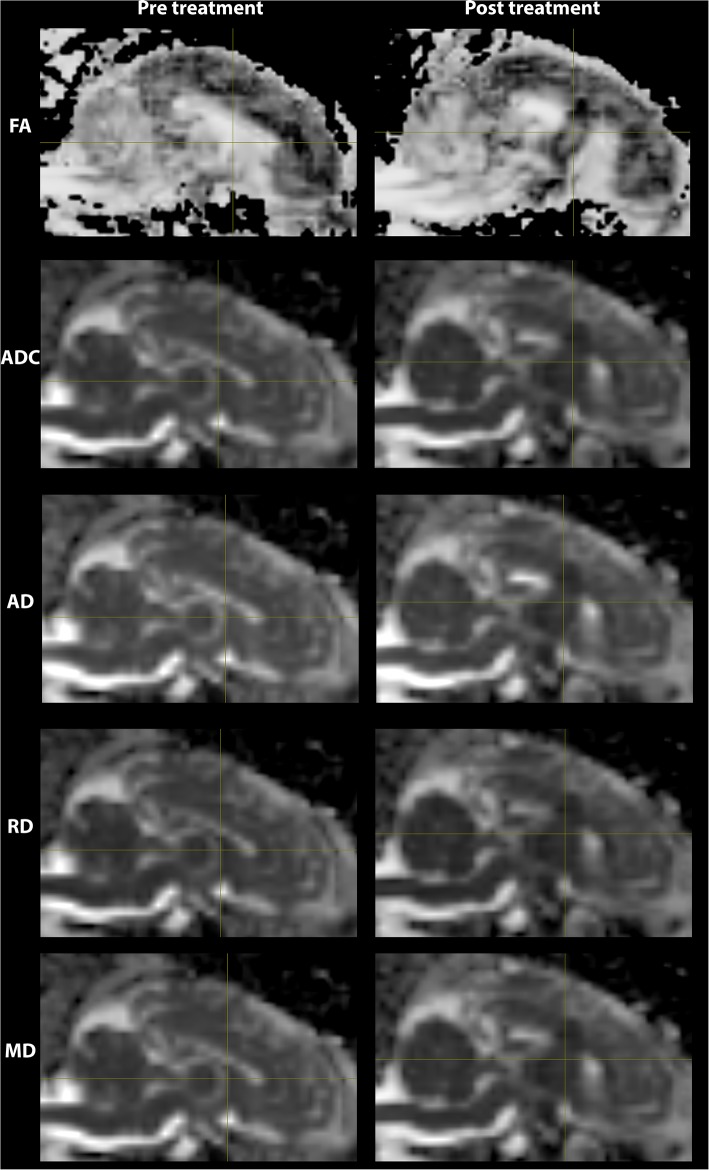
DWI metric maps (FA, ADC, AD, RD, and MD) before and after MRgFUS treatment for one subject. Hypointensity was present in all five metric maps after treatment.
